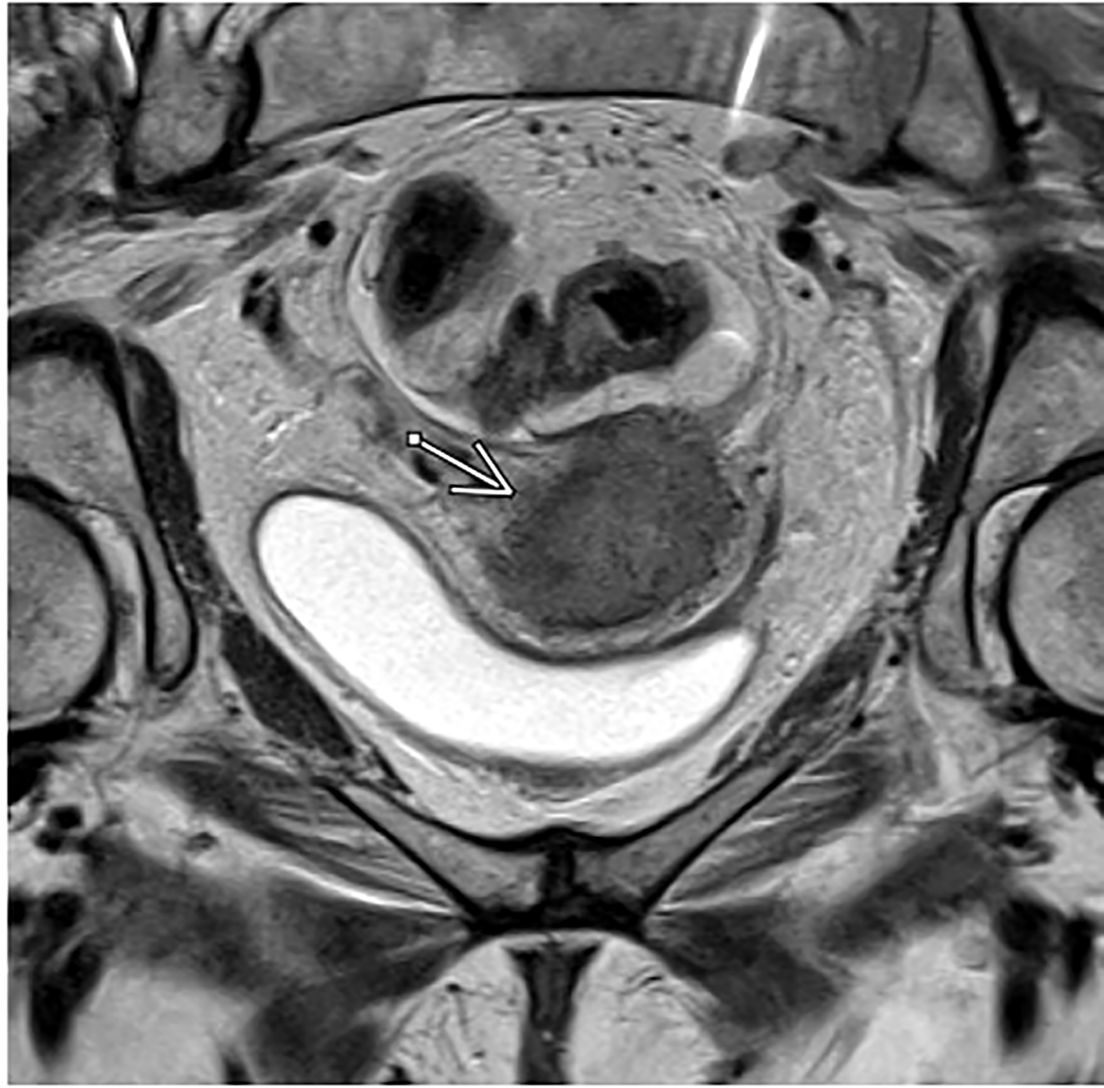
Axial T2-weighted MRI in a patient with FIGO IIIC1, pathologic T2 squamous cell carcinoma of the cervix.
